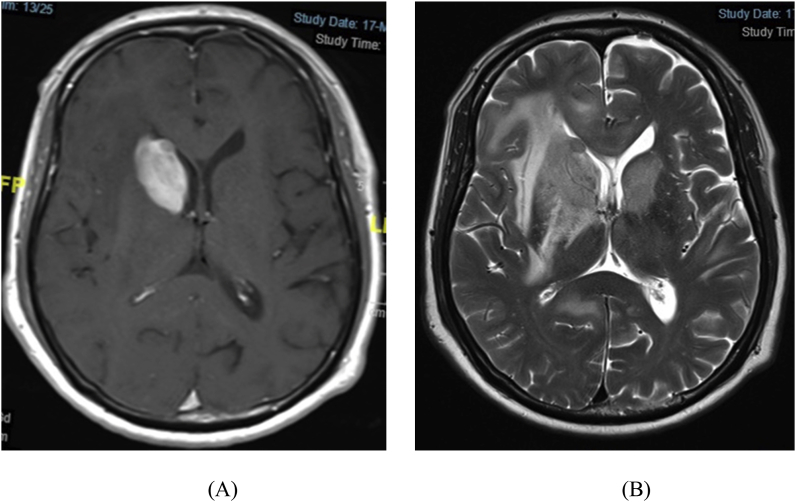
Brain MRI shows vividly enhancing intra-axial mass with irregular margins occupying the head of the right caudate nucleus. Another mass at the right side of the body of the corpus callosum extending cranially to involve the right cingulate gyrus. Significant perilesional edema with local loss of grey-white matter differentiation, no midline shift, slightly compressed right lateral ventricle with no hydrocephalus. T1-Weighted Image (A) and T2-Weighted Image (B).
